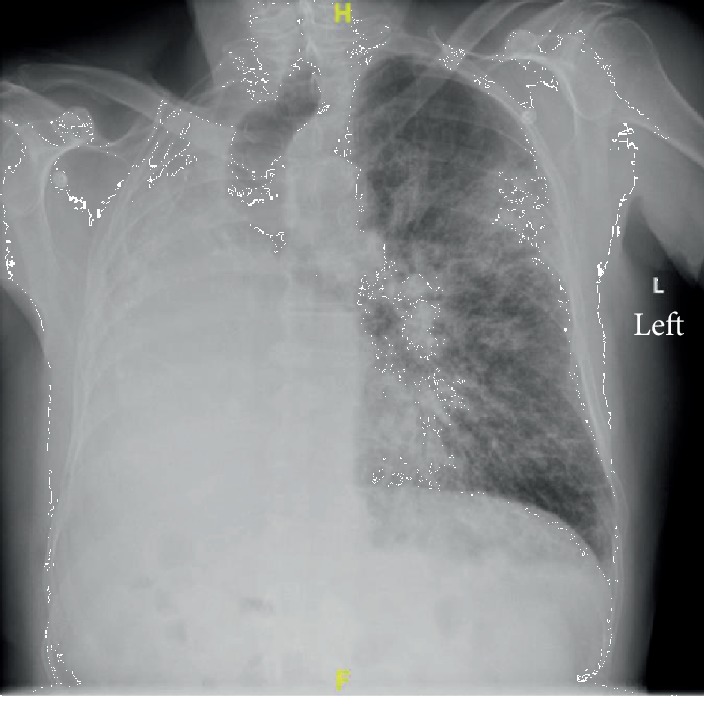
Initial chest X-ray showing complete opacification of the right hemithorax from pneumonectomy. Right mediastinal shift present. Diffuse infiltrates noted in the left lung.
